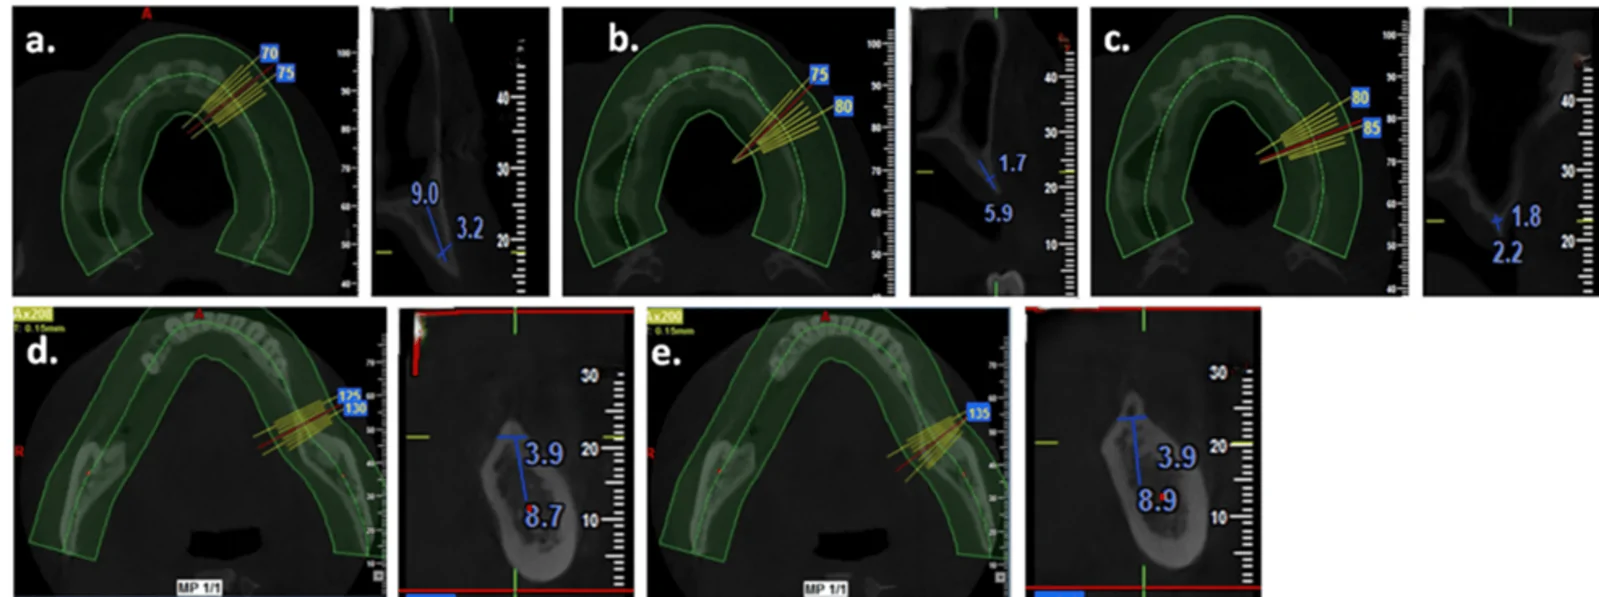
Preoperative cone beam computed tomography assessment of bone dimensions at the proposed implant sites. Preoperative cone beam computed tomography of (a) implant site 23, (b) implant site 25, (c) implant site 27, (d) implant site 36, and (e) implant site 37.
